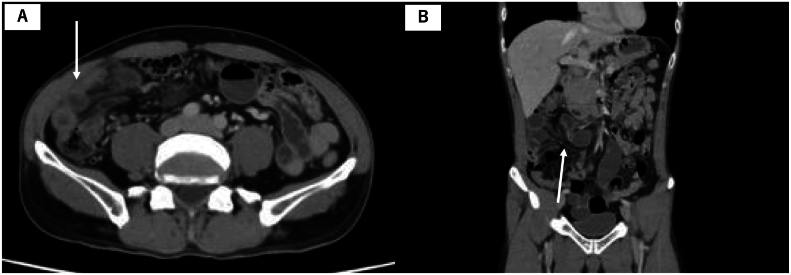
Abdominal enhanced computed tomographic images. A. There are edematous changes in the small intestine (white arrow). B. Vascular convergence and edematous changes are observed in the intestinal mesentery, and a caliber change point of the small intestine is observed nearby (white arrow).
